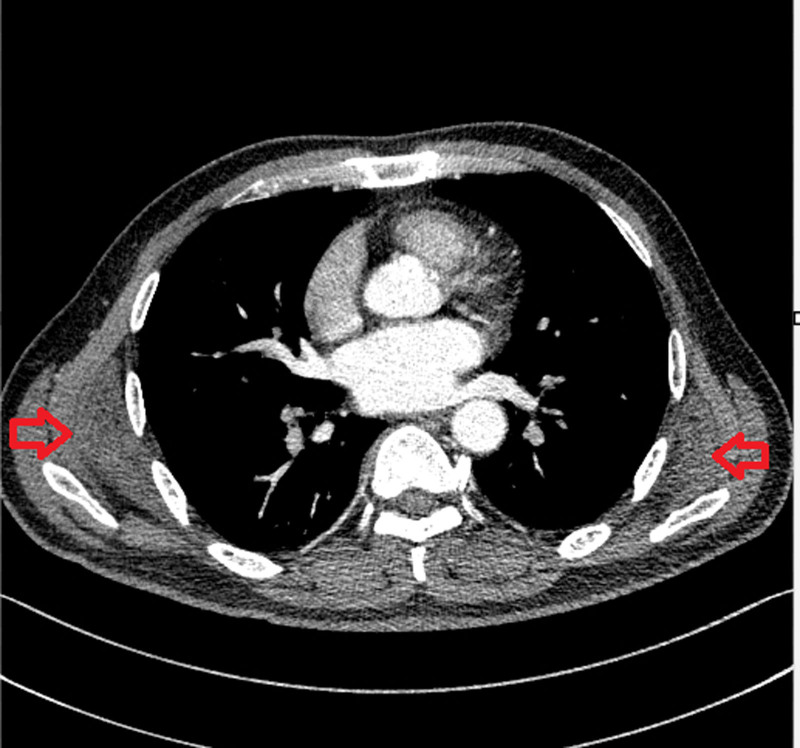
The preoperative CT showed that the density of the masses below the scapula, posterior medial to the serratus anterior muscle, and anterior latissimus dorsi on both sides was slightly low-density, and the density was uniform, similar to the surrounding muscle tissue, and the CT value was about 40 Hu, with blurred boundaries and symmetry. Enhancement scan enhancement is not obvious.
